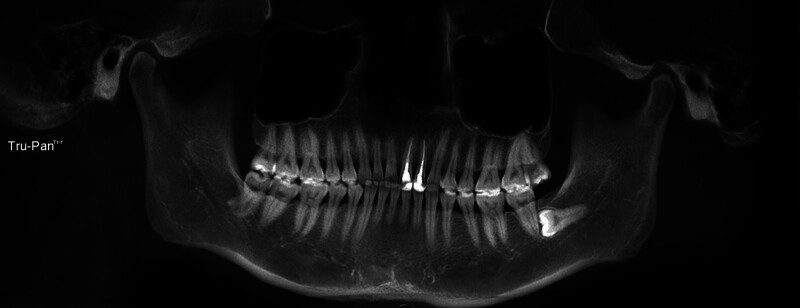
CBCT panorama. CBCT = cone beam computed tomography.
